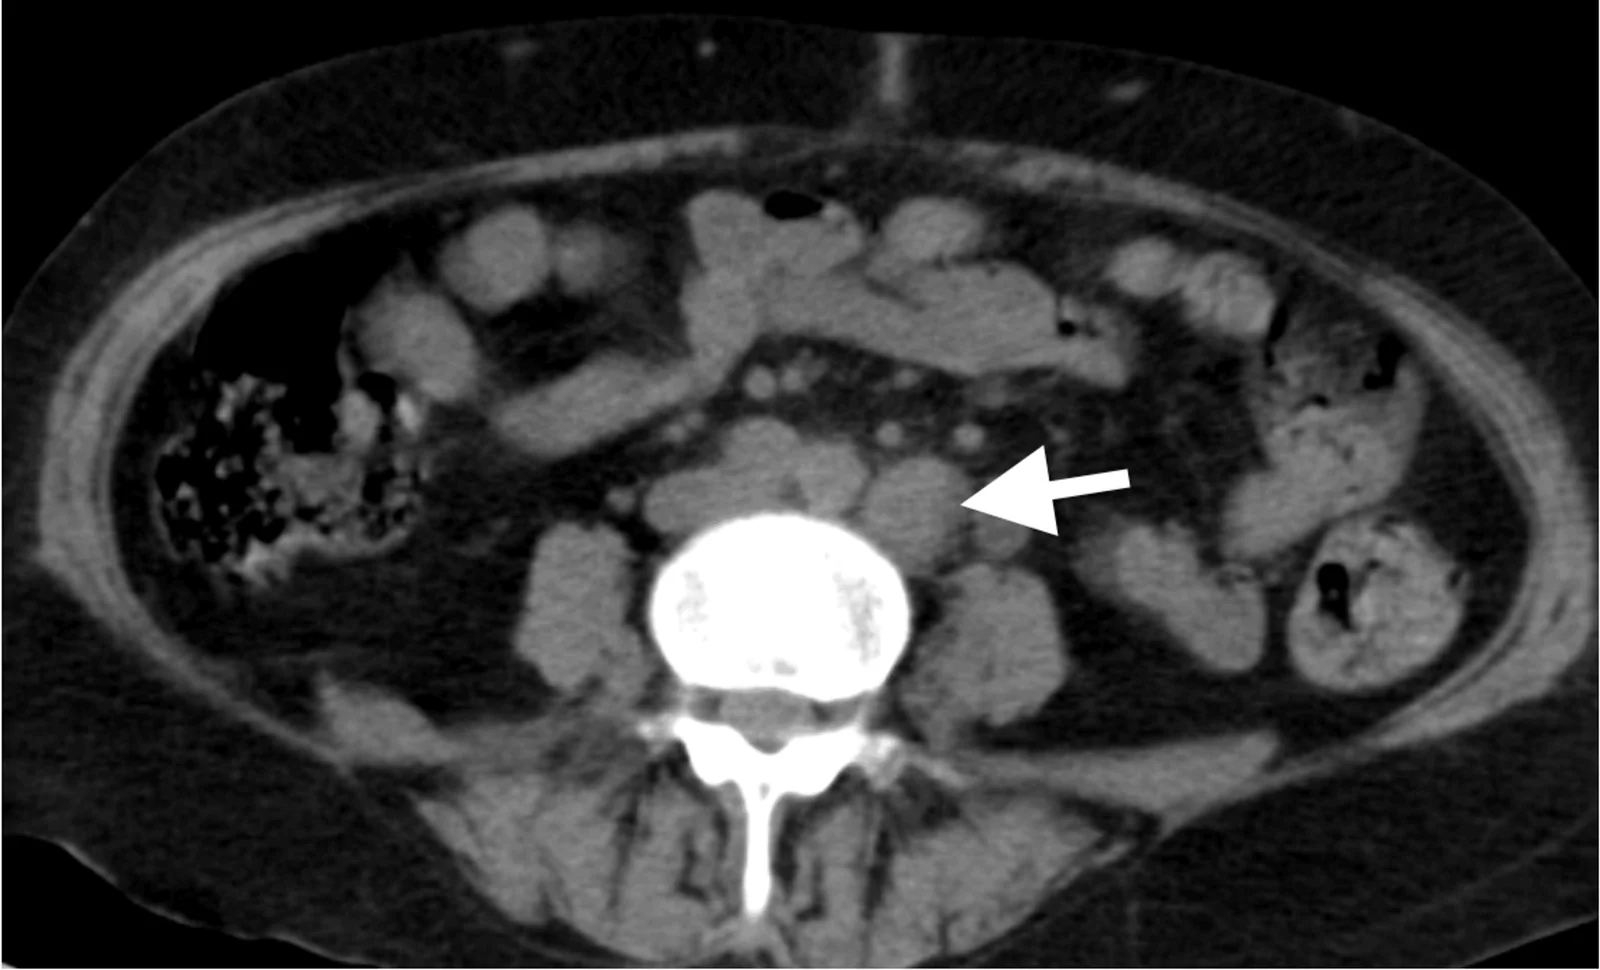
Axial non-contrast CT image of the abdomen showing multiple enlarged retroperitoneal lymph nodes in the preaortic and para-aortic regions (arrow). The image shows multiple enlarged retroperitoneal lymph nodes in the preaortic and para-aortic regions, as indicated by the arrow. In the appropriate clinical context, these findings may be related to an infectious etiology. The largest lymph node measures approximately 1.8 cm in short-axis diameter.
